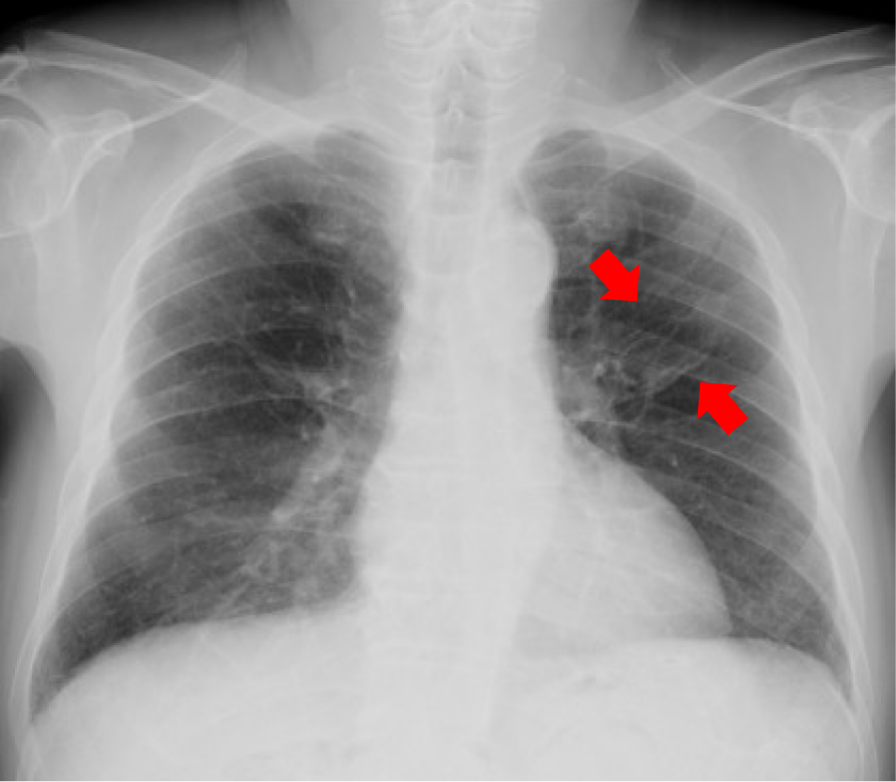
Chest radiographs showing the cavitary lesion (red arrows) in the left middle lung field
